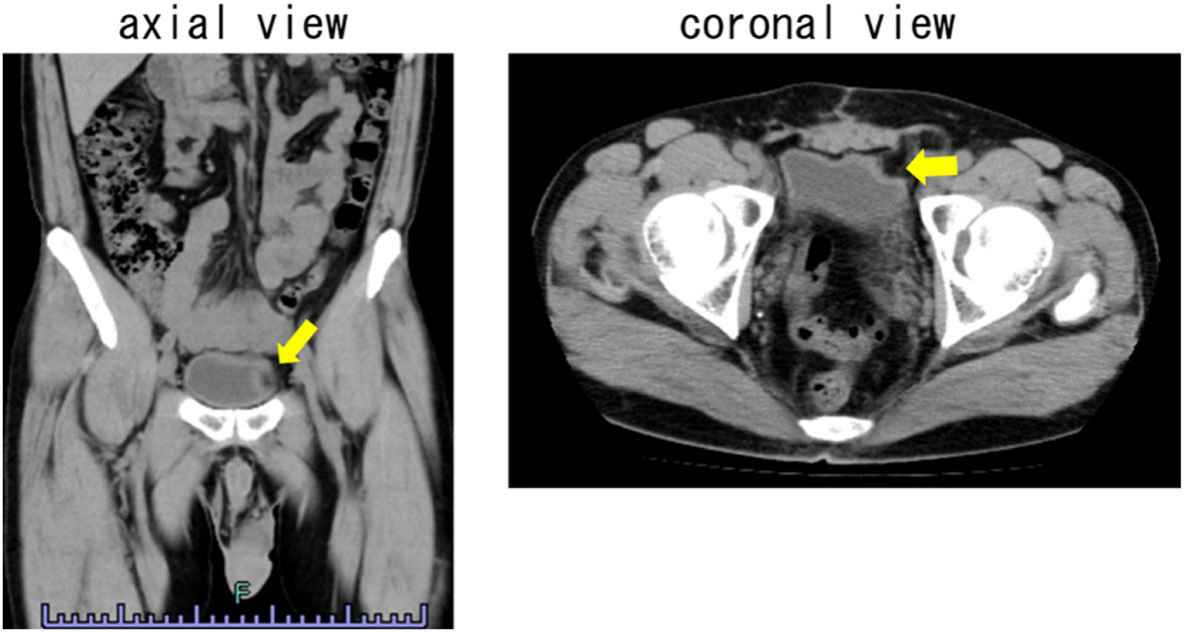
Abdominal CT findings 3 months after the operation showed inversion of the bladder left wall (arrow), but no bowel invagination was observed
